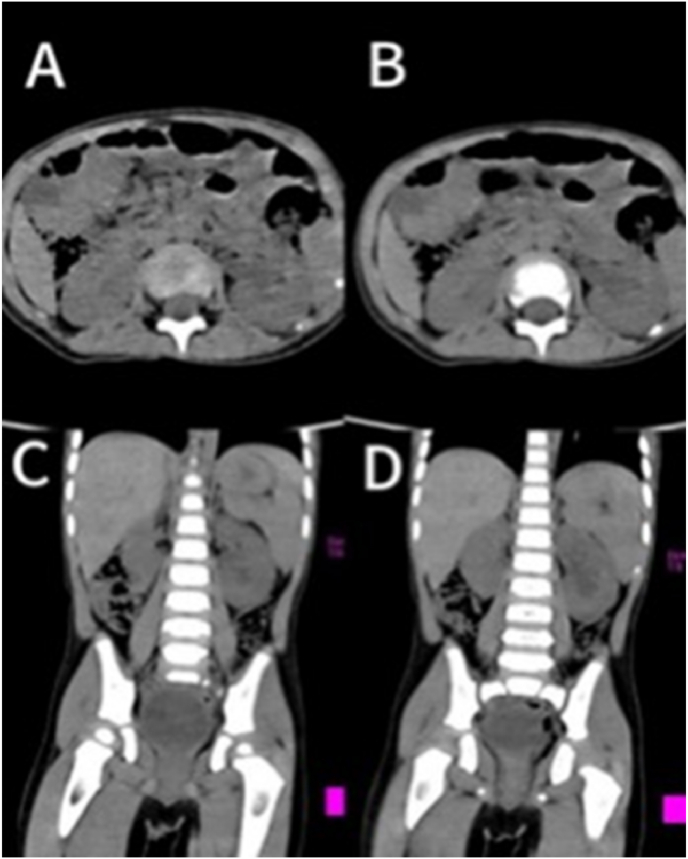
(A–D):plain CT scan of the urinary system revealed hydrodilation of the left renal pelvis and ureter. (A–B): Plain CT scan in the horizontal plane of the urinary system; (C–D): Plain CT scan in the coronal view of the urinary system.
